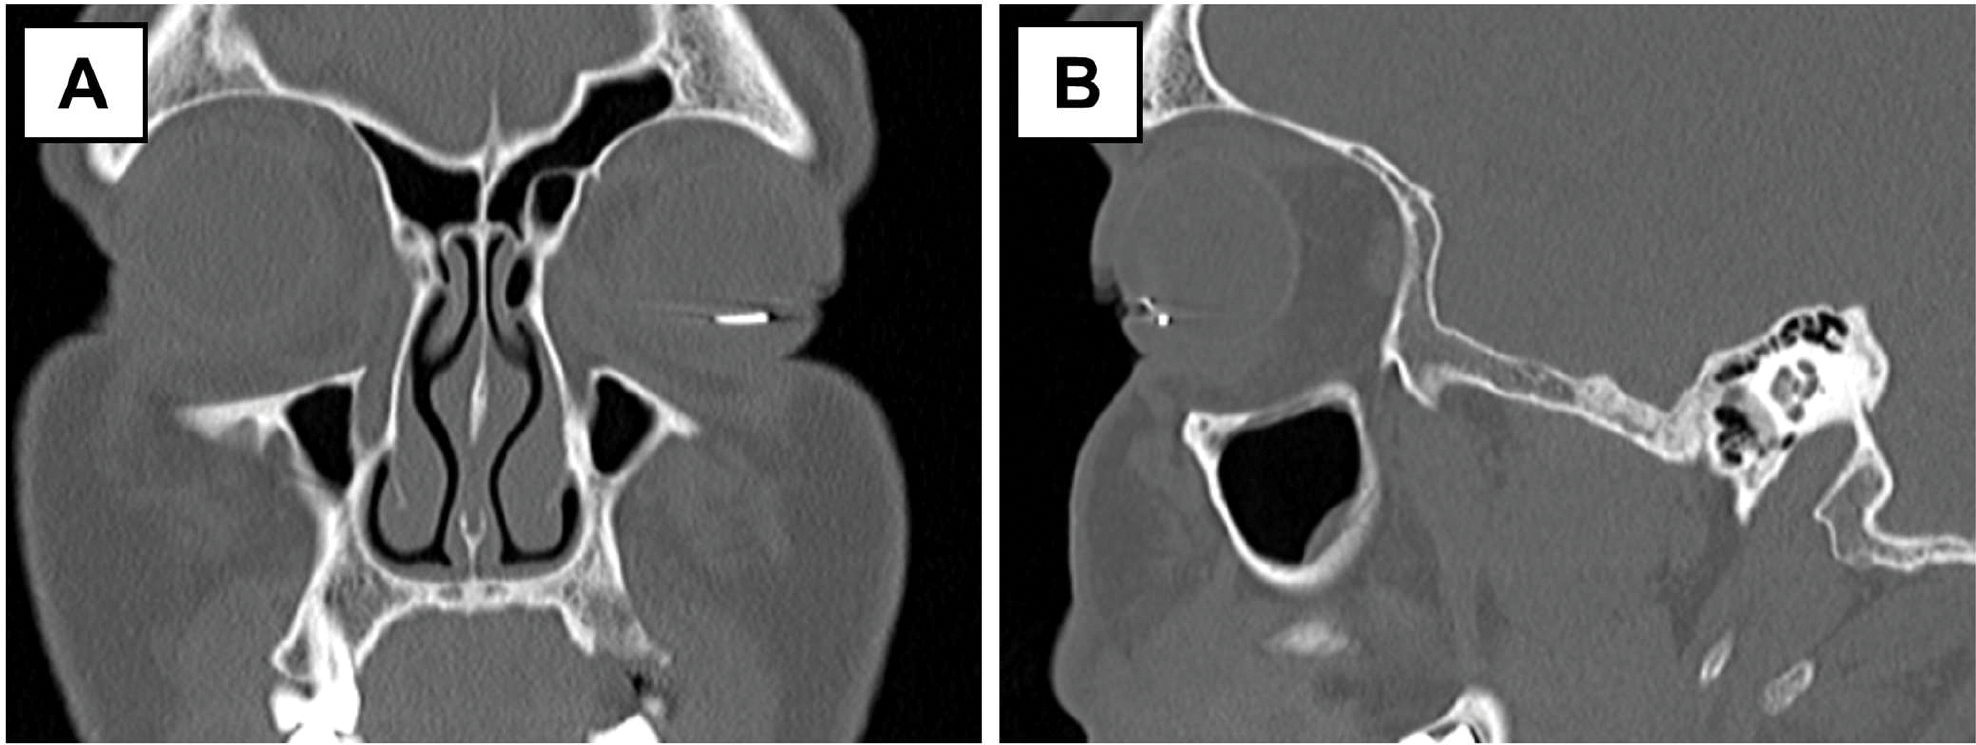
CT scan of the orbit in the coronal (A) and sagittal (B) views demonstrates a hyperintense signal corresponding to the metallic foreign body in the anterior segment of the left eye.
